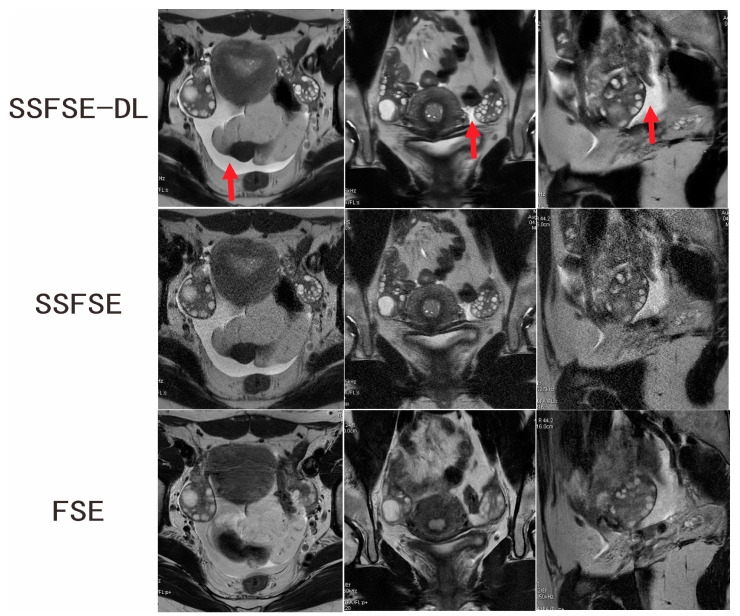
Ovarian MRI in a 16-year-old adolescent girl with confirmed PCOS. SSFSE-DL images (upper row) show the lowest noise, blurring artifacts, and the bilateral enlarged ovaries with a dominant follicle and many small follicles. The pelvic fluid collection (arrow) is easily detected on the SSFSE-DL images. Noise is prominent on the SSFSE images (middle row). The FSE images (lower row) mainly showed blurring artifacts caused by respiratory and bowel motility.
